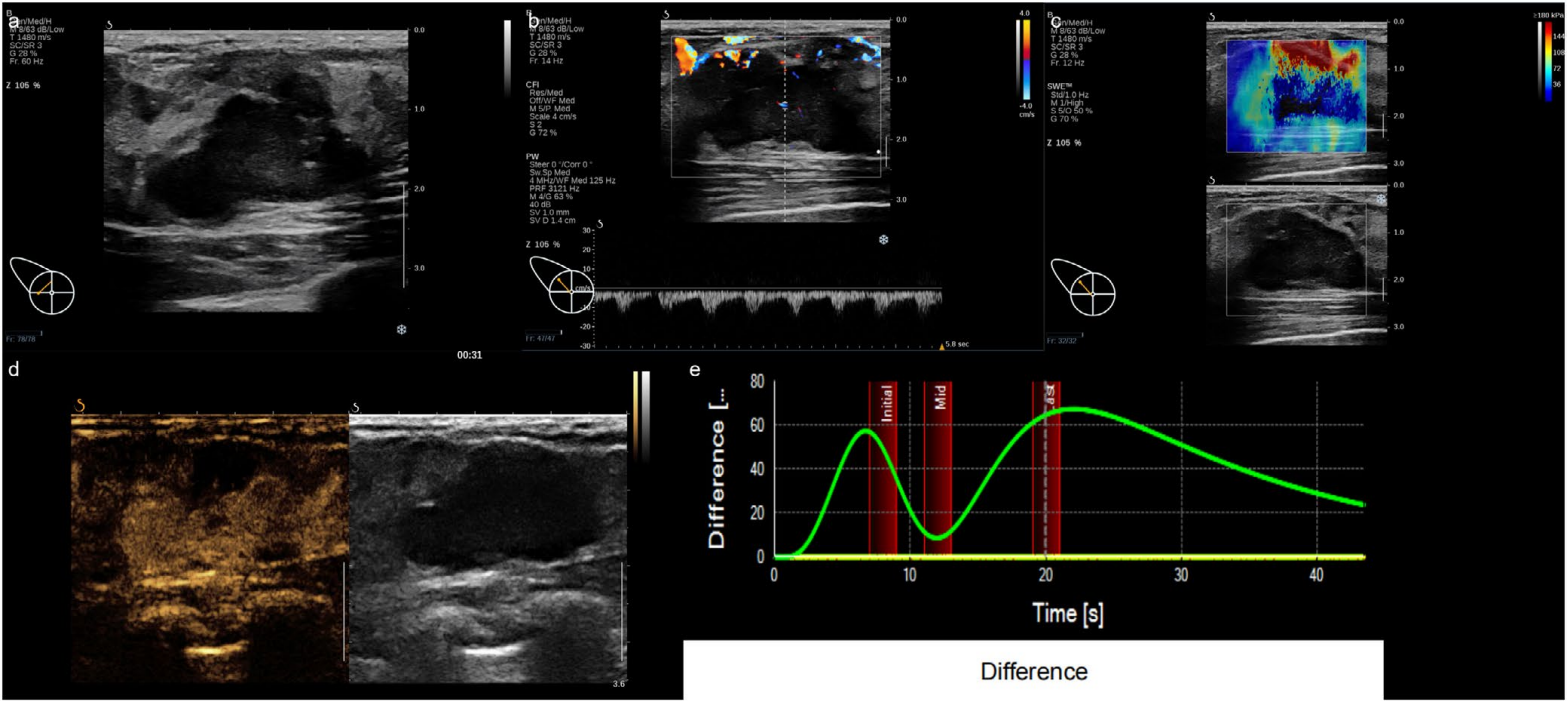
Multimodal ultrasonographic findings in HER‐2 positive breast cancer. The hypoechoic mass displays lobulated margins, calcifications, and Grade III flow. SWE reveals a stiff ring sign. CEUS shows persistent high enhancement with filling defects and a consistently elevated DVPC curve.
